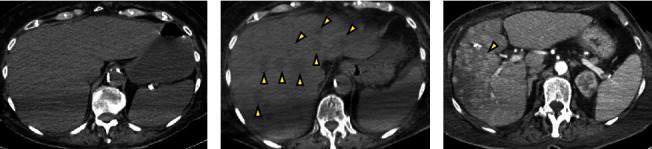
Computed tomography imaging of the abdomen and pelvis without intravenous contrast showing transverse view of (a) homogeneous appearing liver on day 1 with subsequent development of (b) ill-defined hypodense lesions (arrows) with the largest lesion measuring 5.6 cm within the central liver on day 6 and (c) repeat imaging with IV contrast showing progression of disease with innumerable masses at 2 months.
